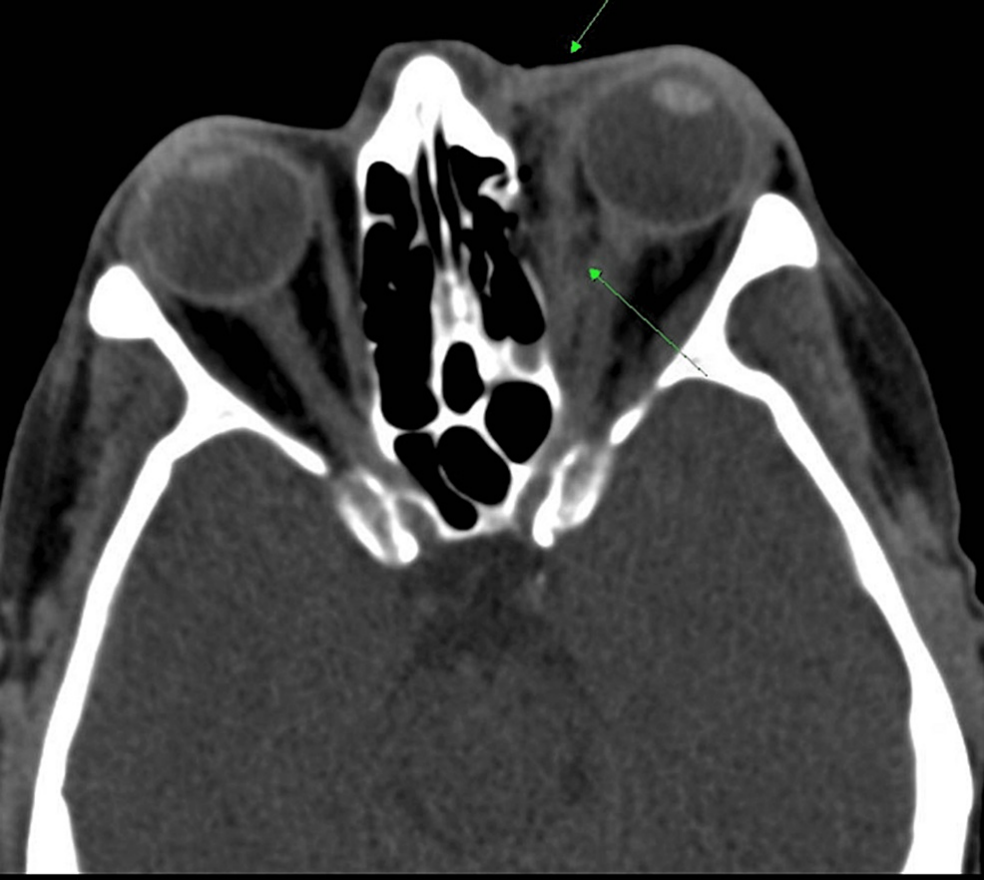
CT of the maxillofacial region reveals stranding of intraorbital fat and periorbital soft tissue swelling of left orbit.
